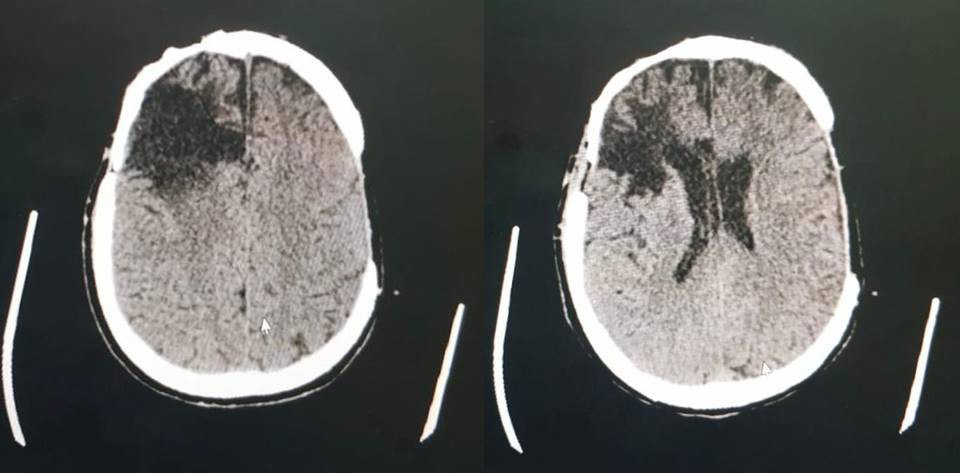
Computed tomography of the head without contrast, showing hypoattenuation of the right cortico-subcortical frontal and left temporal regions and ectasia of adjacent cortical sulci and lateral ventricles, suggestive of encephalomalacia/gliosis.
